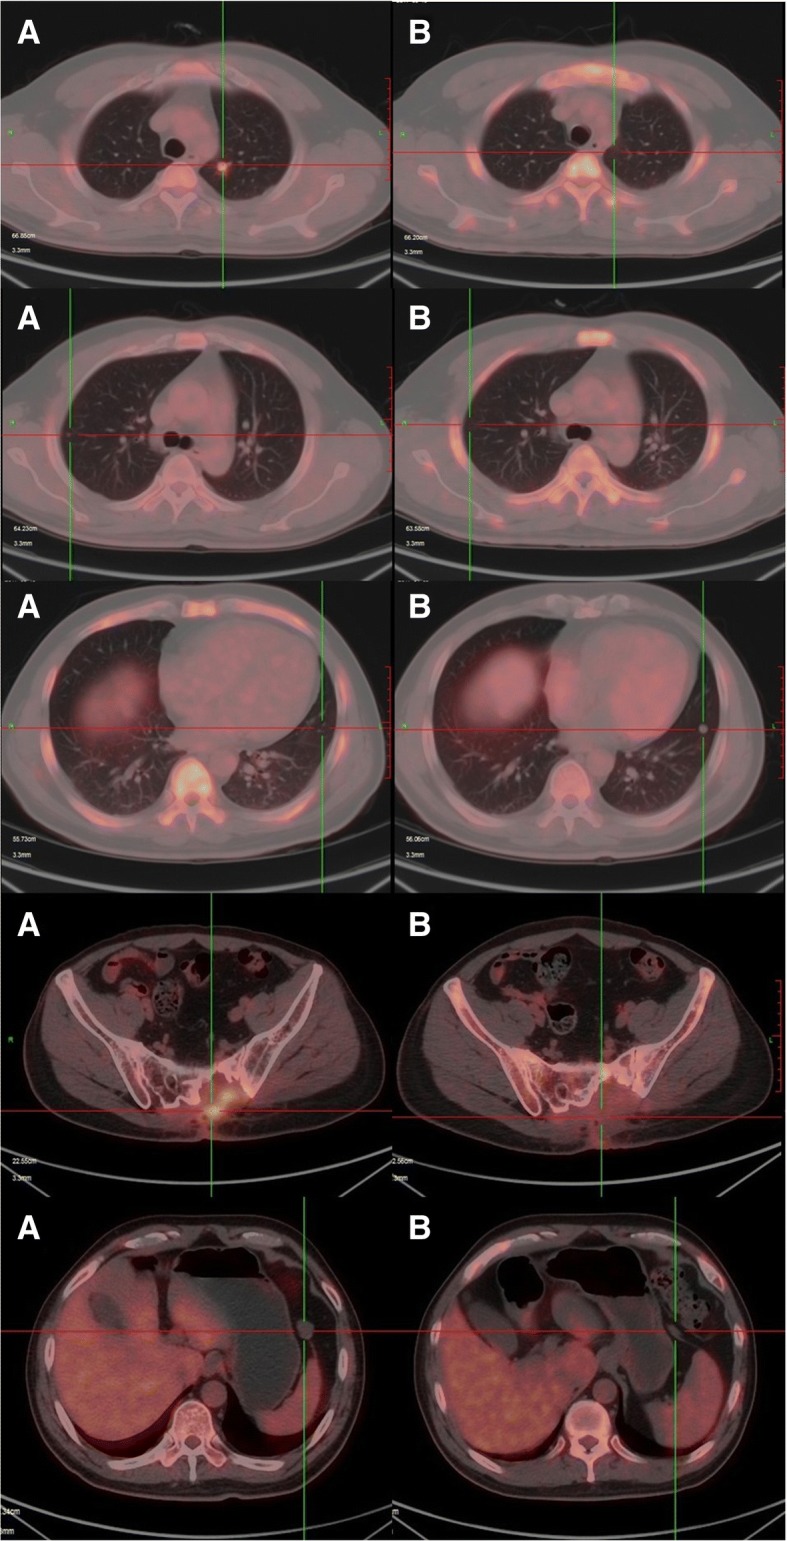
PET-CT images showing visible regression of the multiple metastases after 16 weeks of crizotinib monocherapy. a Follow-up PET-CT image taken on January 10, 2017 at 3 months after surgery showed hypermetabolic metastases in multiple regions, before the start of cizotinib treatment. b Follow-up PET-CT images taken on May 19, 2017 at 4 months after initiation of crizotinib showed near-CCR
